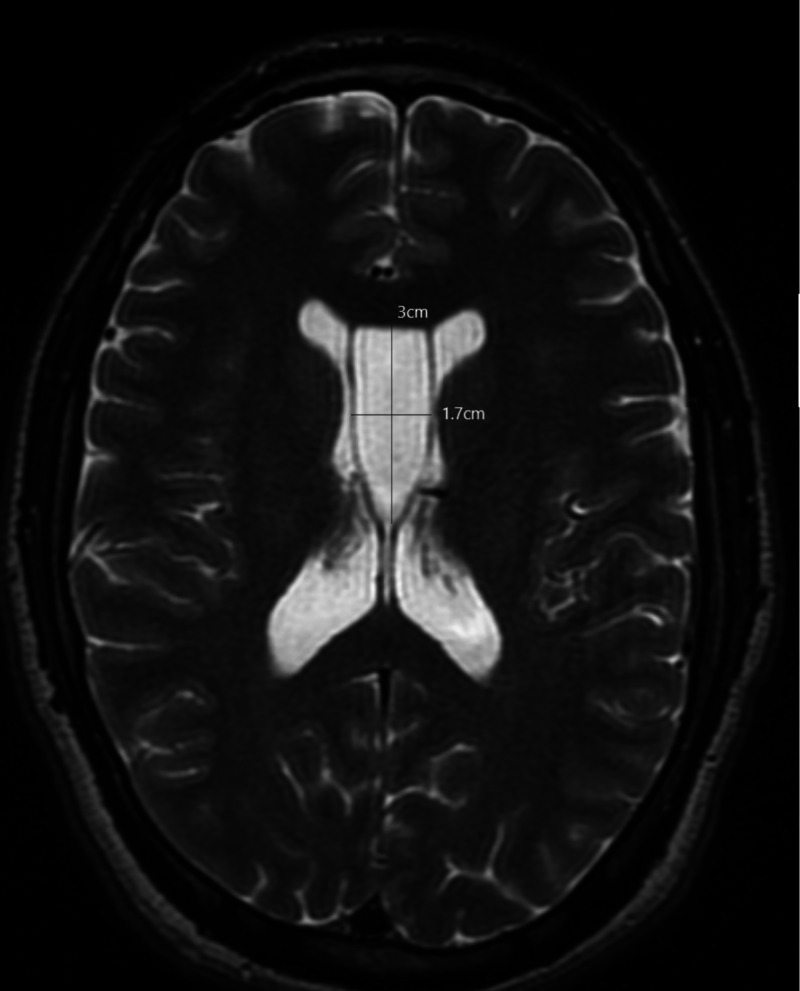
T2 weighted magnetic resonance imaging (MRI) of the head in axial view This is a T2 weighted magnetic resonance imaging (MRI) of the head in axial view that is showing the anteroposterior extent of the cyst of the cavum septum pellucidum along with the cavum vergae. The 3 X 1.7 cm cyst is appreciable in this section with definite bowing of the lateral walls.
